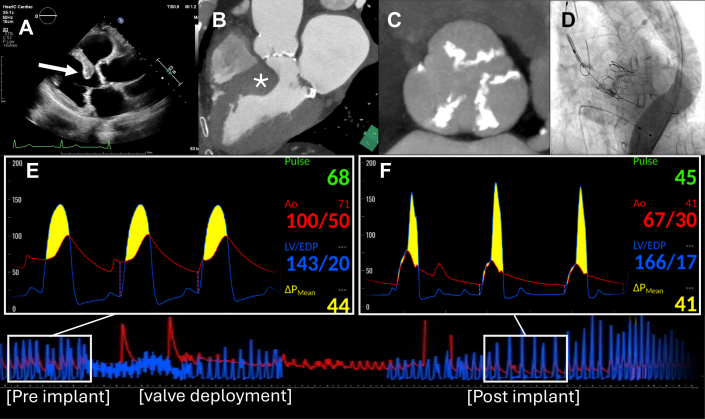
Detecting LVOT Obstruction Using Continuous Pressure Monitoring With the SavvyWire During TAVI (A and B) TTE and CT demonstrating the interventricular septal bulge. (C) All cusps of the aortic valve are severely calcified. (D) TAVI was performed, with the prosthesis delivered using the SavvyWire, while continuously monitoring LV pressure. (E) Before valve implantation, the SavvyWire showed an elevated LV pressure. Aortic pressure exhibited a lower peak compared to LV pressure, confirming the presence of severe aortic stenosis. (F) After valve implantation, a marked drop in aortic pressure was observed, and the SavvyWire revealed a further significant increase in LV pressure and a late systolic peaking of the pressure waveform. These findings indicated acute LVOT obstruction. CT = computed tomography; LV = left ventricle; LVOT = left ventricular outflow tract; TAVI = transcatheter aortic valve implantation; TTE = transthoracic echocardiography.
